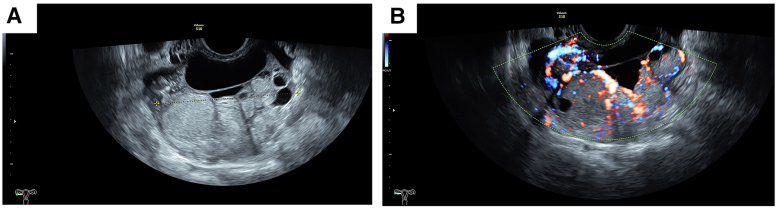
Pelvic ultrasound image. A solid mass in the right adnexal region (A), with abundant blood flow within it (B).
